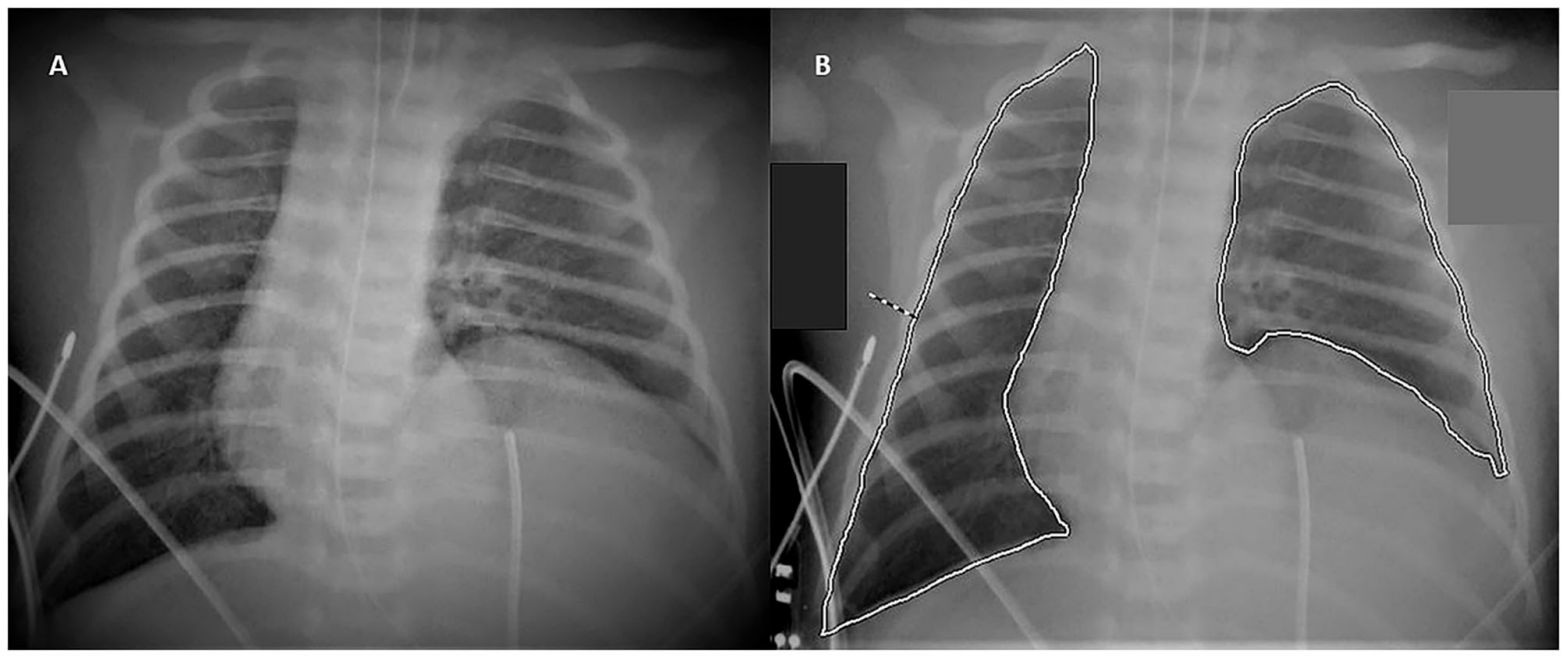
Tracing Method. The figure shows the method to calculate the area of the lungs: chest radiograph of a 1-day-old infant without (A) and with (B) the freehand tracing of the perimeter of the lungs.
